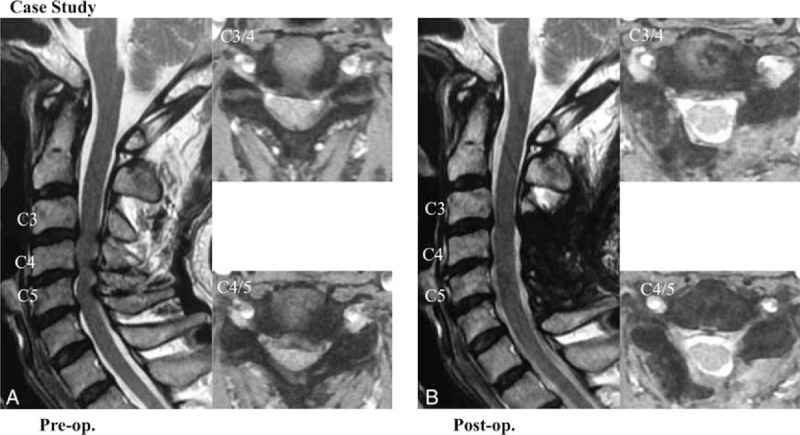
Preoperative (A) and postoperative (B) T2-weighted sagittal MR imaging in a 72-year-old man with multilevel cervical spondylotic myelopathy.
